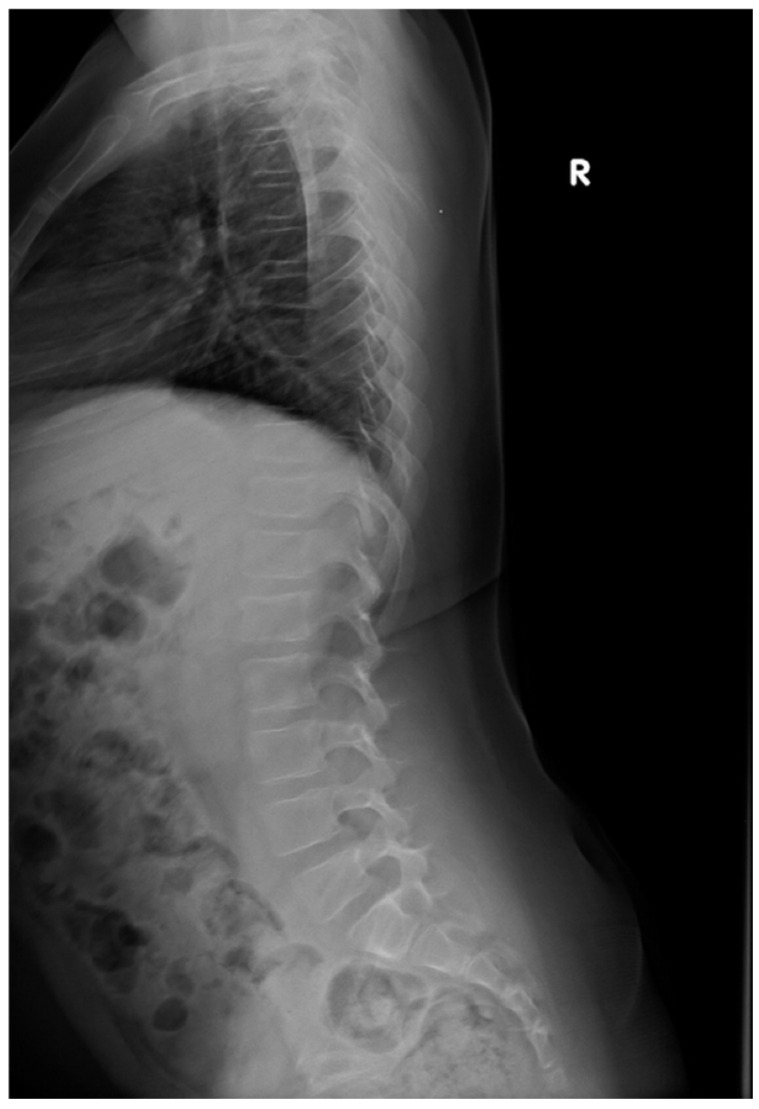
X-ray image of Patient 1 showing fractures in the vertebrae Th7, Th11, and L1.
